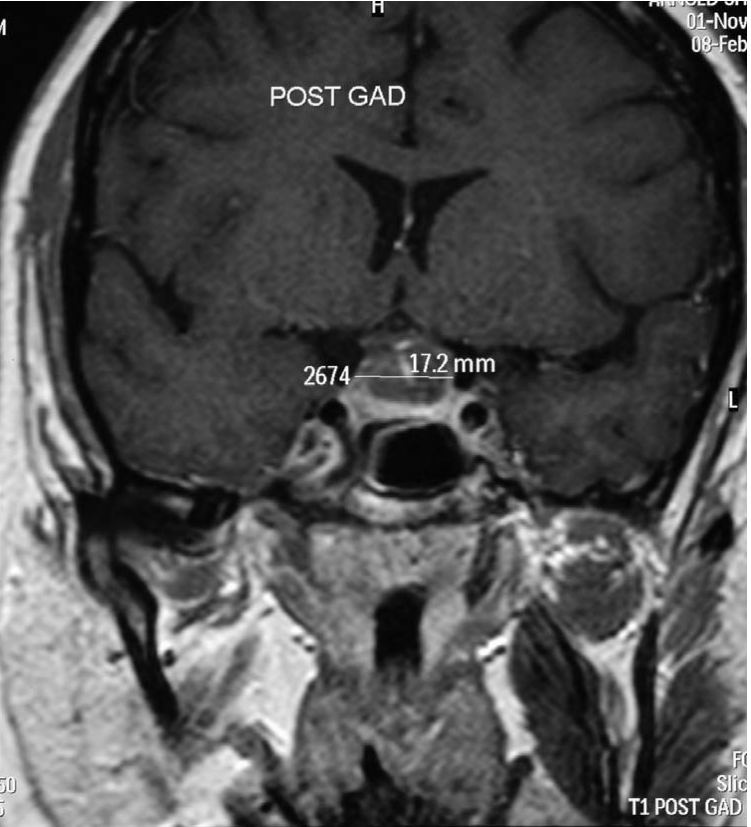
Magnetic resonance T1-weighted contrast-enhanced imaging; coronal view.
